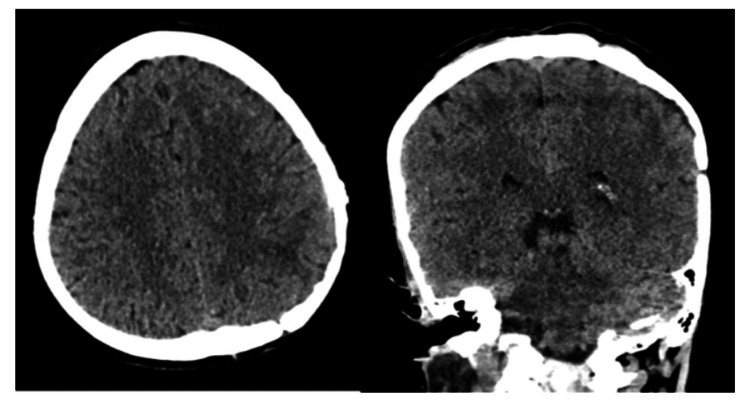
Computed tomography (CT) scan of the brain Six-month postoperative follow-up images confirmed no recurrence.
